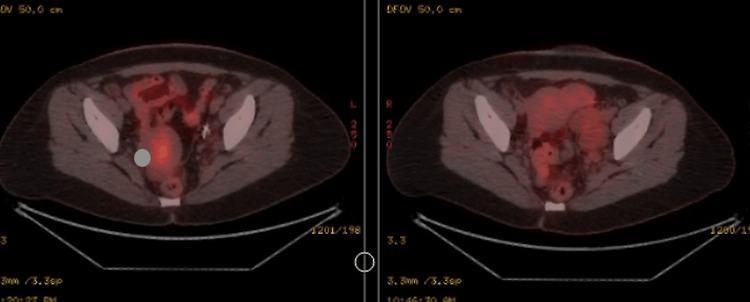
PET/CT imaging of retroperitoneal angiomyolipoma (PEComa) Axial fused PET/CT images demonstrate a well-circumscribed retroperitoneal mass (left panel, oval shape) located adjacent to the left adnexa, showing mild FDG uptake. The right panel shows interval postoperative imaging with stable postoperative changes and no new hypermetabolic lesions. PEComa - perivascular epithelioid cell tumor
